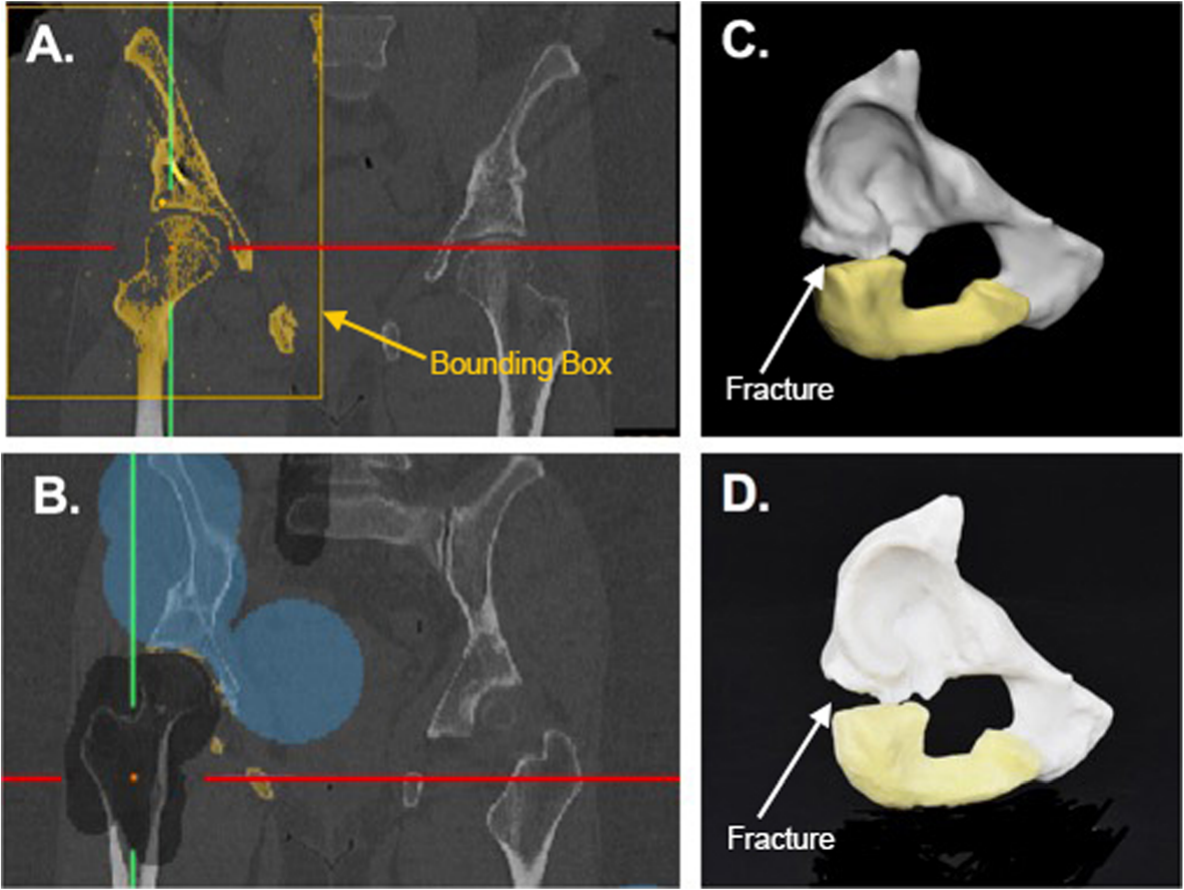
a Coronal CT image showing threhsolded right pelvic bones, showing similar colors for the pubis, ischium, and femur. b Coronal CT image showing splitting of the pelvis (blue) from the femur (black). c 3D computer model showing the pubis (white) and ischium (yellow). d Photograph of 3D printed model
